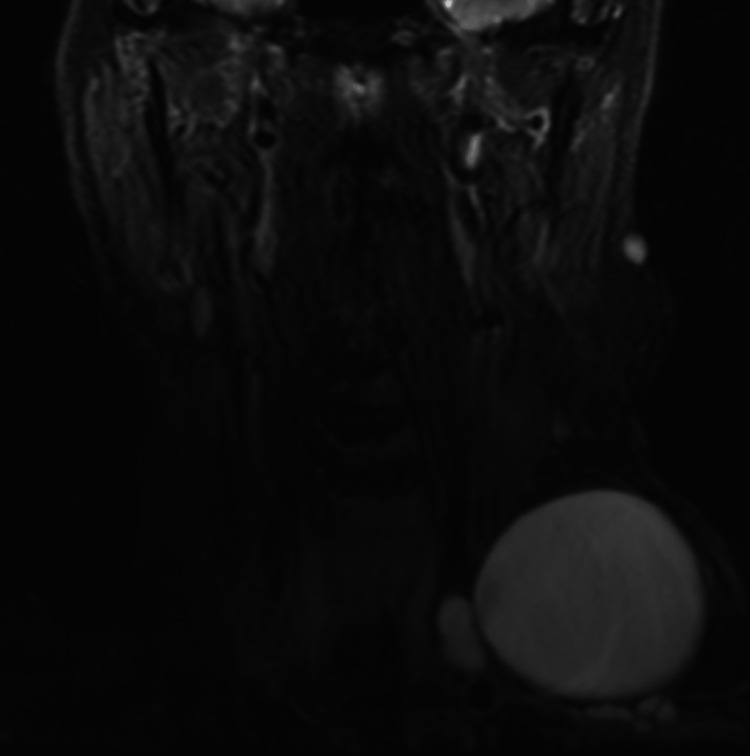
Coronal MRI of head and neck T2 coronal MRI of patient's head and neck area. There is a sizeable left-sided neck mass that is hyperintense on T2 with serpiginous channels located just deep to the dominant mass, suggestive of a cervical lymphocele.
